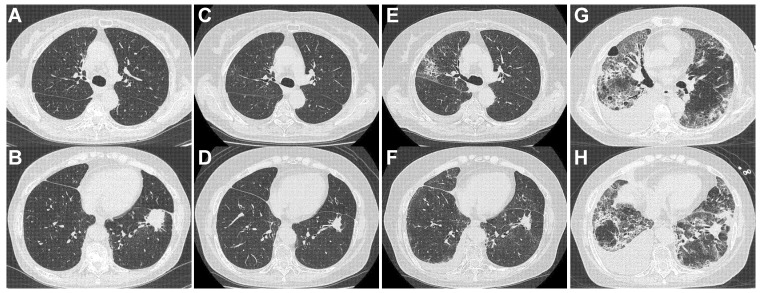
Case 1, a 65-year-old female. CT images. (A,B) Before pembrolizumab. (C,D) After 13 cycles of pembrolizumab. (E,F) After 22 cycles of pembrolizumab. (G,H) After an additional 3 months of corticosteroid therapy.
